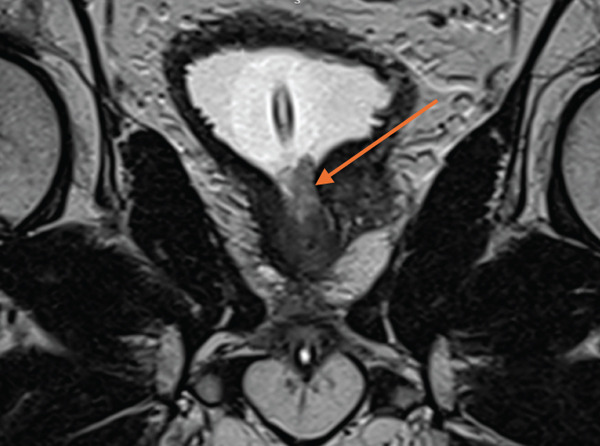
Coronal section of T2‐weighted MRI image showing a non‐enhancing lesion in the prostatic urethra, adjacent to the catheter and extending into the bladder neck, marked with an arrow.
